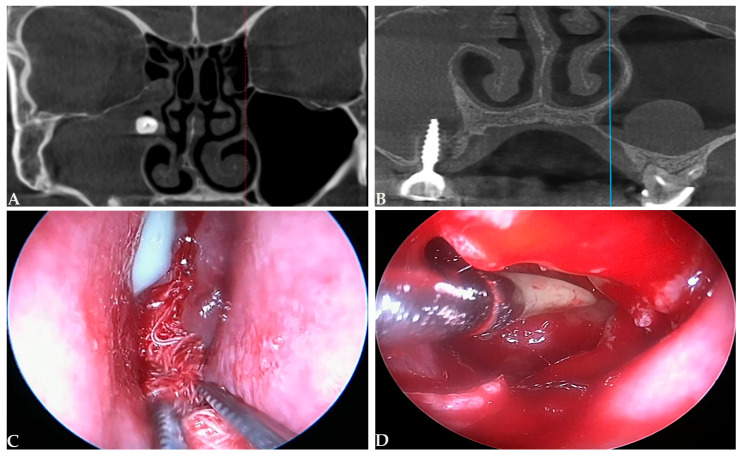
Iatrogenic odontogenic sinusitis induced by dental procedures. CT scans showing maxillary intrasinusal root (A) and implant (B); Endoscopic sinus surgery showing pus drainage (C) and root elimination (D). (Photos from the personal archive of the author SA).
